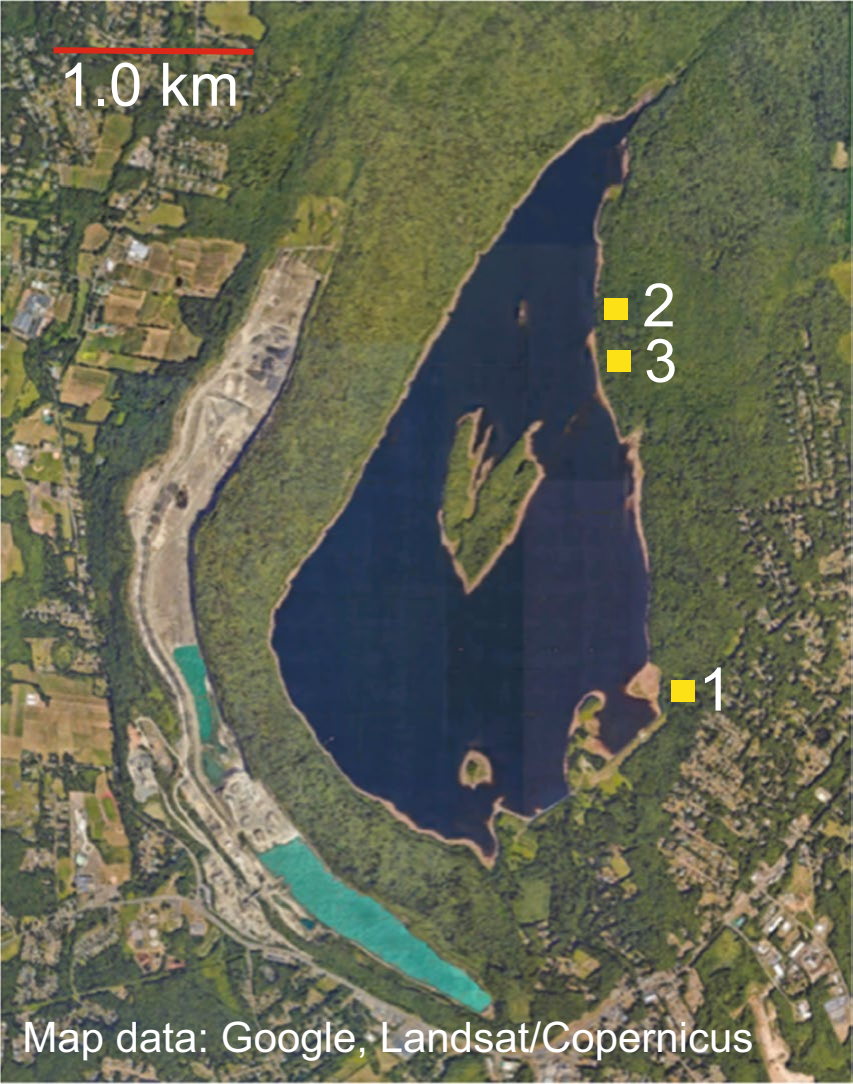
Aerial photograph of mixed hardwood forest field site at Lake Gaillard, CT in June 2018. Numbers indicate the locations of trapping sites 1–3 for capture-and-release of *P. leucopus*. The GPS coordinates for center of the lake are 41.347 N 72.783 W. Eye altitude is approximately 10,000 m. Photograph obtained with Google Earth Pro.
